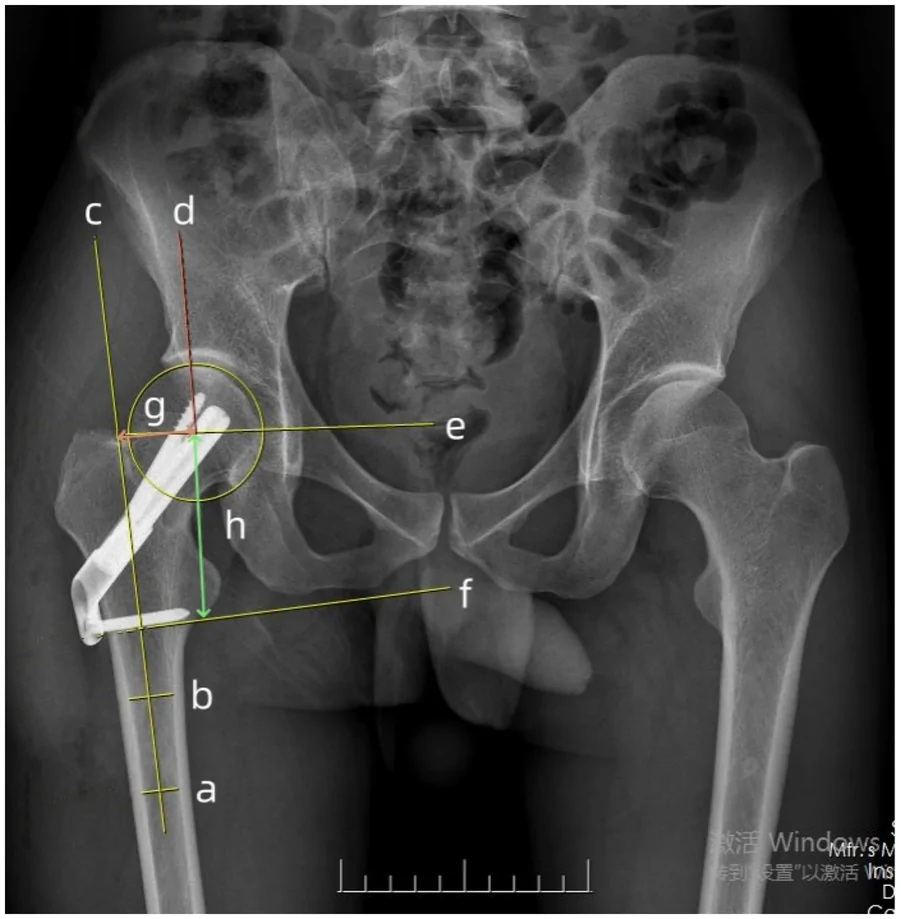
Measurement of femoral neck shortening and femoral offset after femoral neck fracture fixation. Representative radiographic measurements of femoral neck shortening and femoral offset after surgical treatment of femoral neck fractures. FO, femoral offset.
